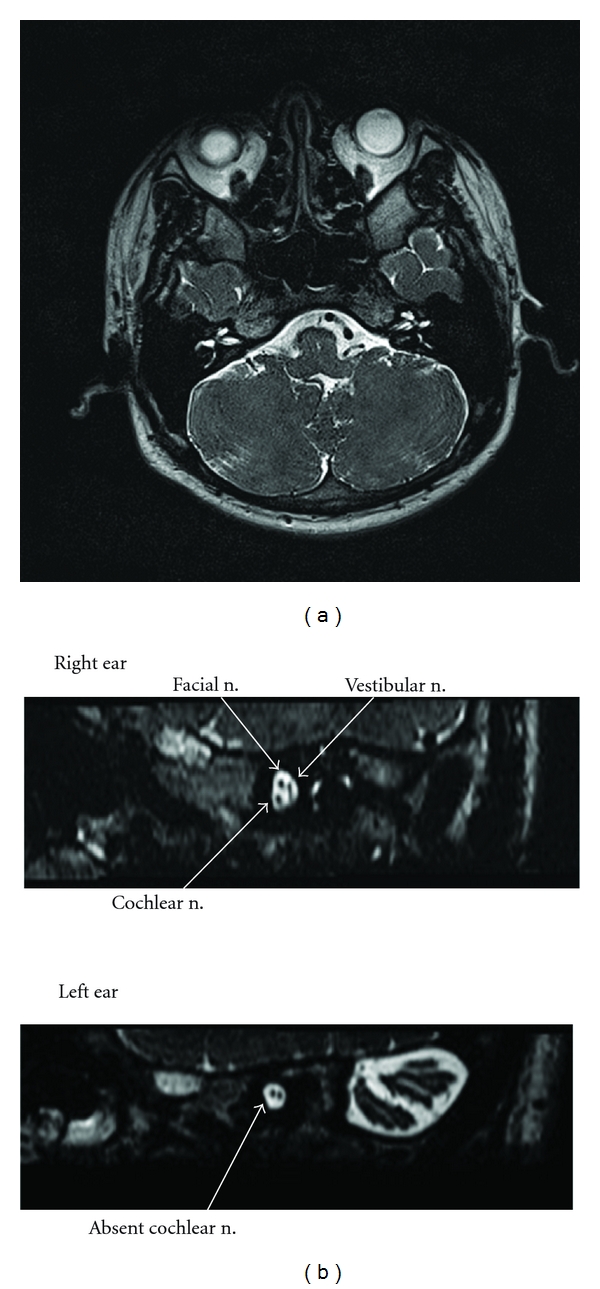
Axial 3-dimensional T2-weighted images through the internal auditory canal of case 1. The reconstructed sagittal oblique images were demonstrated. The right ear has a normal-size cochlear nerve as marked by arrow, while the left cochlear nerve is absent although facial nerve and vestibular nerve have their distinguishable locations.
